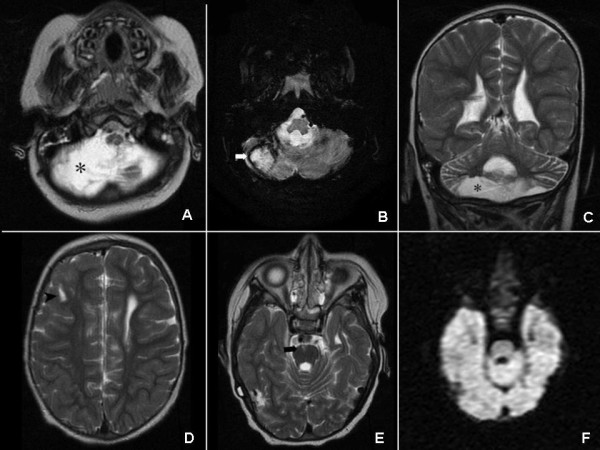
MRI at 3 y. 4 mo. of age. Axial spin echo T2 WI (A), axial gradient echo T2 WI (B) and coronal T2 weighted (C) sections showing chronic bilateral cerebellar haemorrhagic infarctions as encephalomalacia and loss of parenchyma (asterisks). Note hemosiderin (white arrow). D, E: Axial spin echo T2 images showing chronic-appearing lacunar infarctions in subcortical white matter of the right frontal lobe (short black arrow) and peripheral pons (black arrow). No osmotic demyelination features in central pontine fibers. F: Axial diffusion weighted image reveals no restricted diffusion in central pons.
